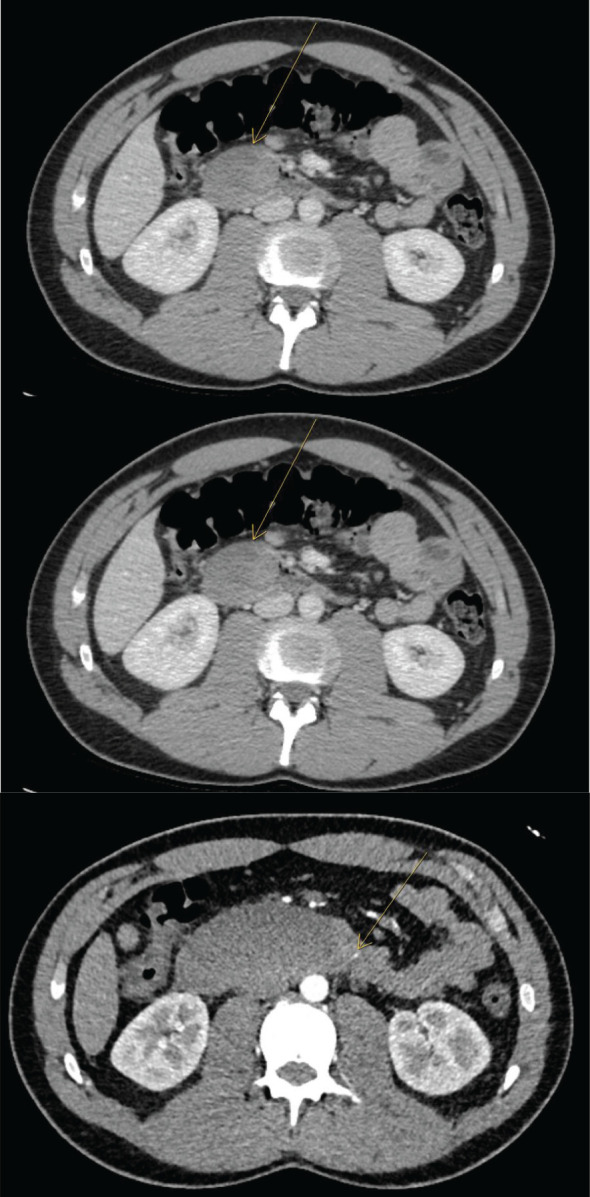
Progressive CT A/P images at day 1 and day 3 with expansion of duodenal hematoma and CT angio at day 5 with area of active extravasation.
